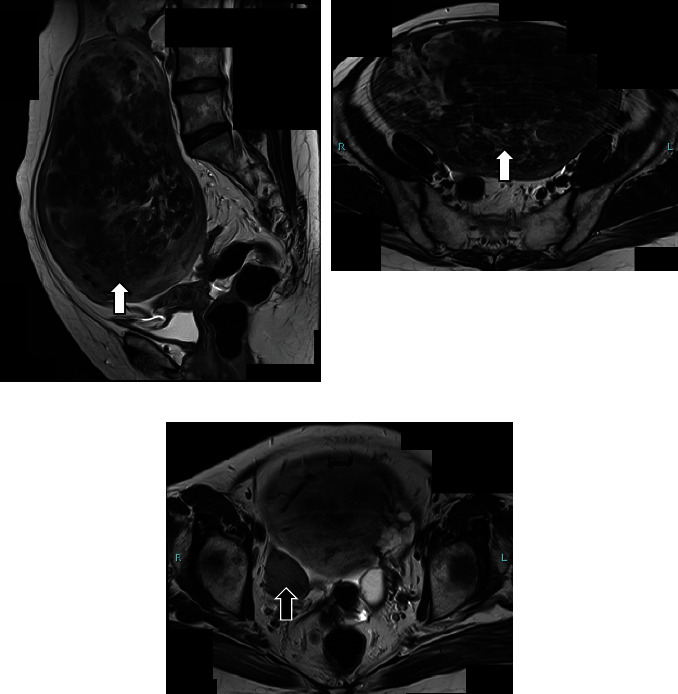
MRI findings on T2-weighted imaging. MRI shows two solid pelvic masses. (a) White and (b) black arrows indicating the different masses.
